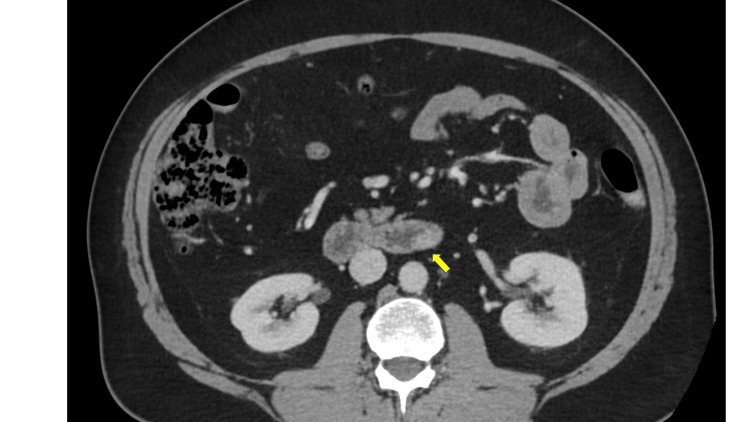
Case 1: Axial CT view of ectopic pancreas. Contrast-enhanced CT of the abdomen, axial reconstruction, demonstrating a well-circumscribed, approximately 2 cm eccentric soft tissue lesion (yellow arrow) arising from the posterior wall at the junction of the third and fourth parts of the duodenum (D3/D4). The lesion projects into the lumen without evidence of obstruction or surrounding inflammatory change and was radiologically suspicious for a GIST. CT: computed tomography; GIST: gastrointestinal stromal tumour
